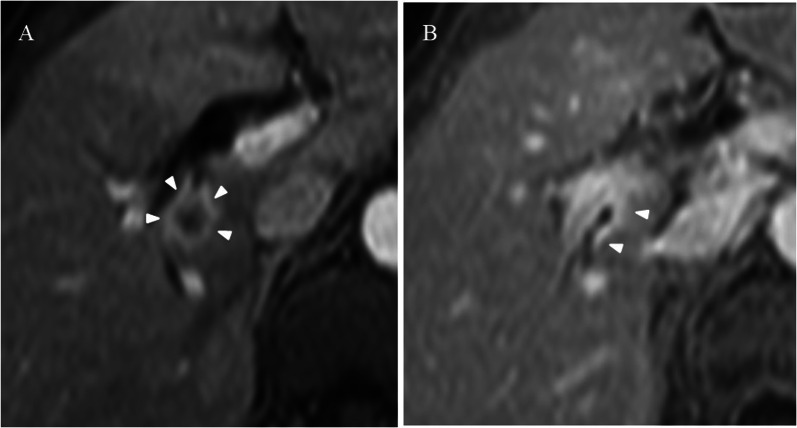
MRI findings. A In the axial section of contrast-enhanced T1-weighted images in the early phase, an irregular circumferential ring-enhancing mass was observed at the right hepatic hilum (arrowheads). B In the axial section at a level of the posterior glissonean confluence, marked wall thickening with enhancement was noted at the bifurcation of the posterior glissonean pedicle (arrowheads)
